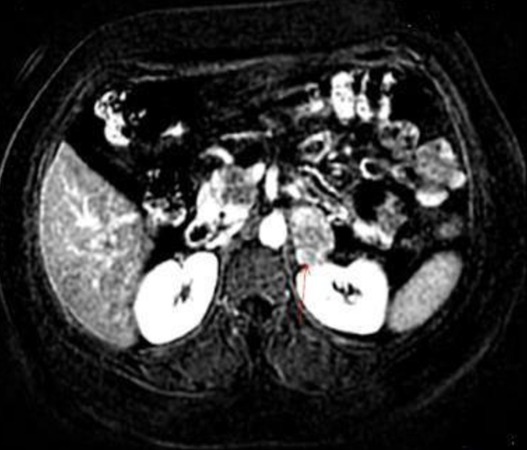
Magnetic resonance imaging revealed a round mass in the left adrenal gland. The mass registered low-signal intensity on the T1-weighted image, and a slightly high signal intensity on the T2-weighted image
